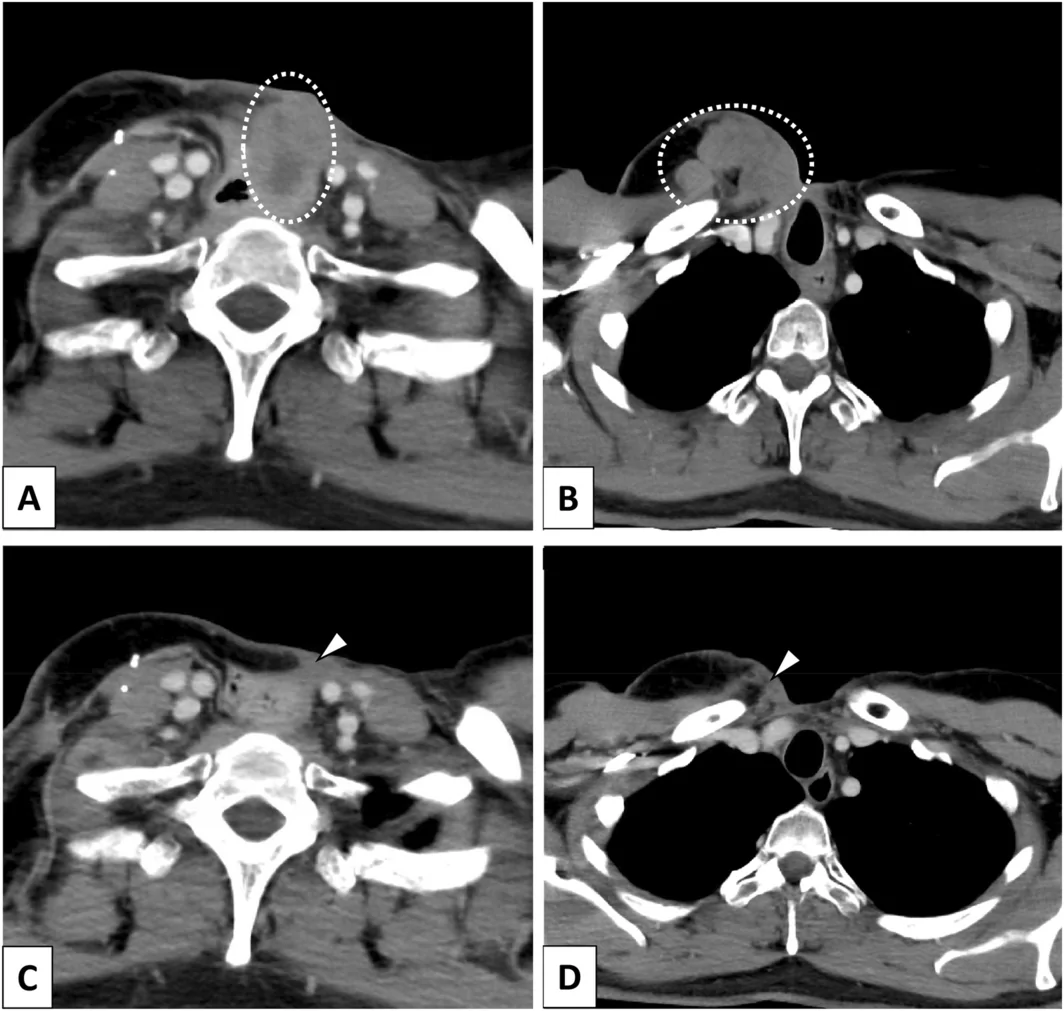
Therapeutic response to BET inhibitor therapy. Contrast‐enhanced CT images obtained in January 20X3 prior to initiation of BET inhibitor therapy. (A) Tumor infiltration into the wall of the free jejunal graft (white dotted circle). (B) Multiple metastatic lesions within and surrounding the deltopectoral flap (white dotted circles). Contrast‐enhanced CT images obtained 3 months after initiation of BET inhibitor therapy demonstrate marked tumor regression, consistent with a partial response. (C, D) Arrows indicate lesions corresponding to those shown in (A) and (B), respectively. BET, bromodomain and extra‐terminal; CT, computed tomography.
